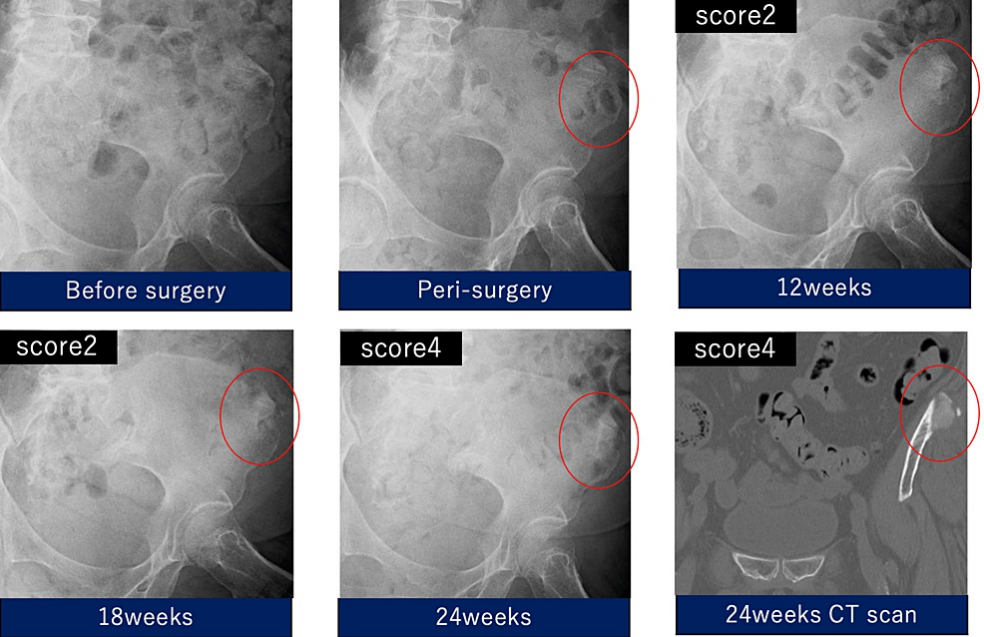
Radiographic evaluation of implanted ORB-03 as an iliac bone graft. The circled area was filled with blood containing ORB-03 for the harvested site of ilium and imaged preoperatively and at 12, 18, and 24 weeks after surgery.
